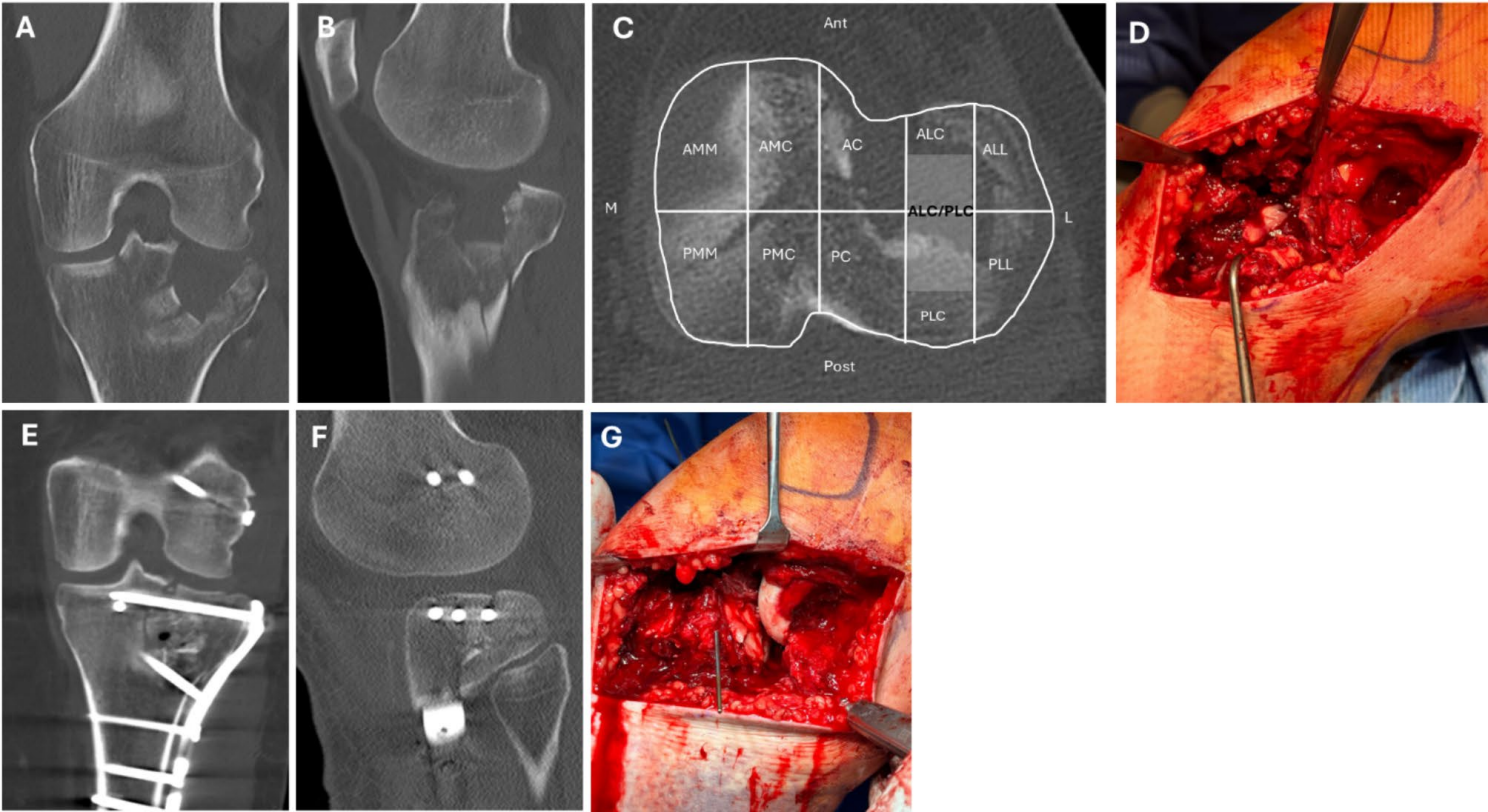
Exemplary cases of a patient treated with lateral epicondyle Osteotomy. A 47-year-old female with an AO/OTA type C3 fracture, involving all segments except the anteromedio-medial (AMM) segment, as defined by the 10-segment classification. The hatched area indicates the crossing of the anterior-lateral column (ALC) and posterior-lateral column (PLC). Preoperative CT scans demonstrate the fracture pattern in coronal (A), sagittal (B), and axial (C) views. Postoperative CT scans show successful reduction and allogenous bone grafting of the articular surface in coronal (E) and sagittal (F) views. Intraoperative images include the fracture prior to reduction after epicondyle osteotomy (D) and temporary fixation with K-wires (G)
